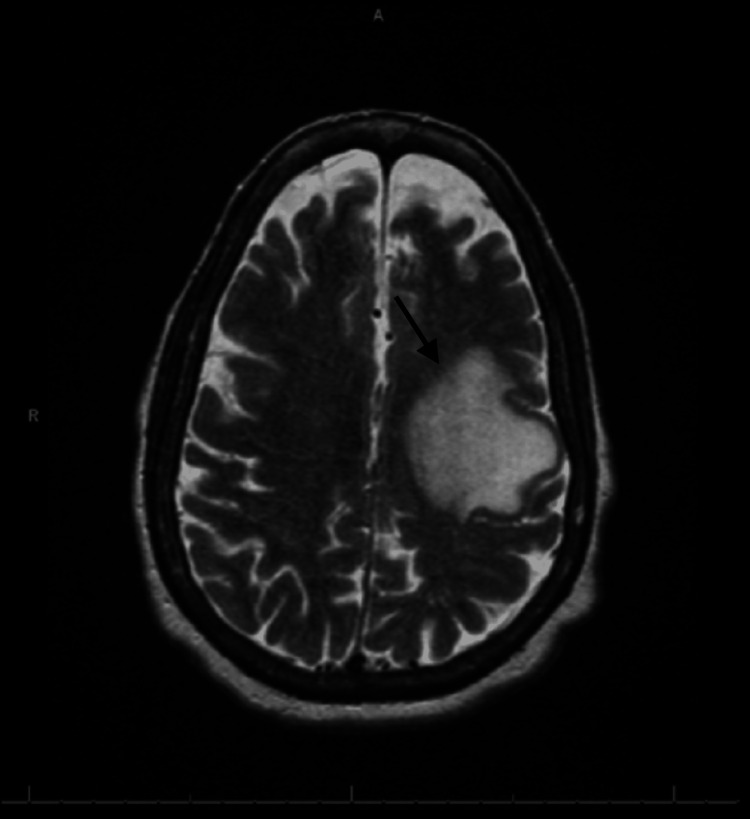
Magnetic resonance imaging of the brain with contrast revealed a lesion in the left frontal lobe with surrounding vasogenic edema and mass effect.
